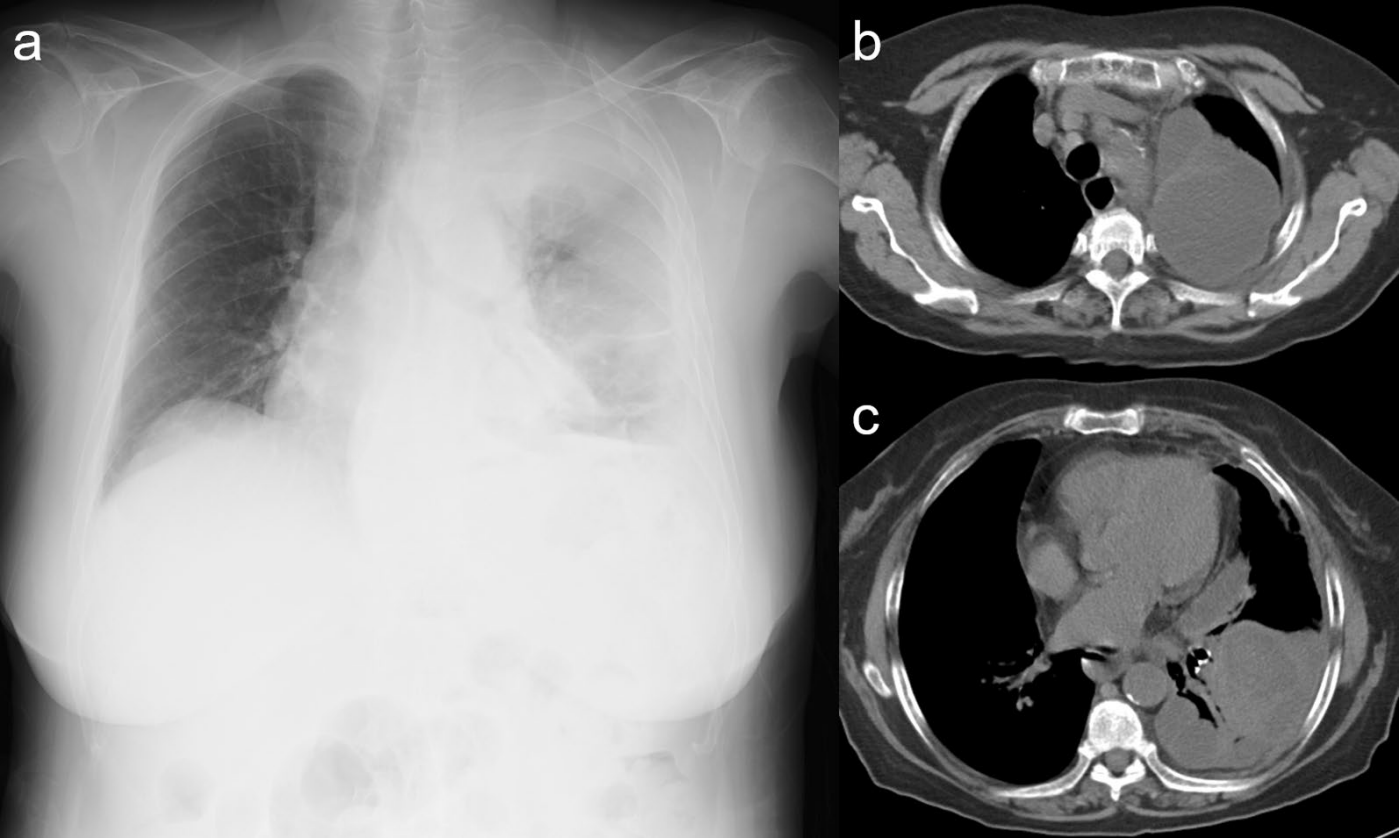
Chest radiography and computed tomography (CT) during the first visit. a Chest radiograph revealing collapse of the left lung and pleural effusion. Chest CT image showing, b multiple cavitated left pleural effusions and (c) a lung abscess in the left lower lobe
